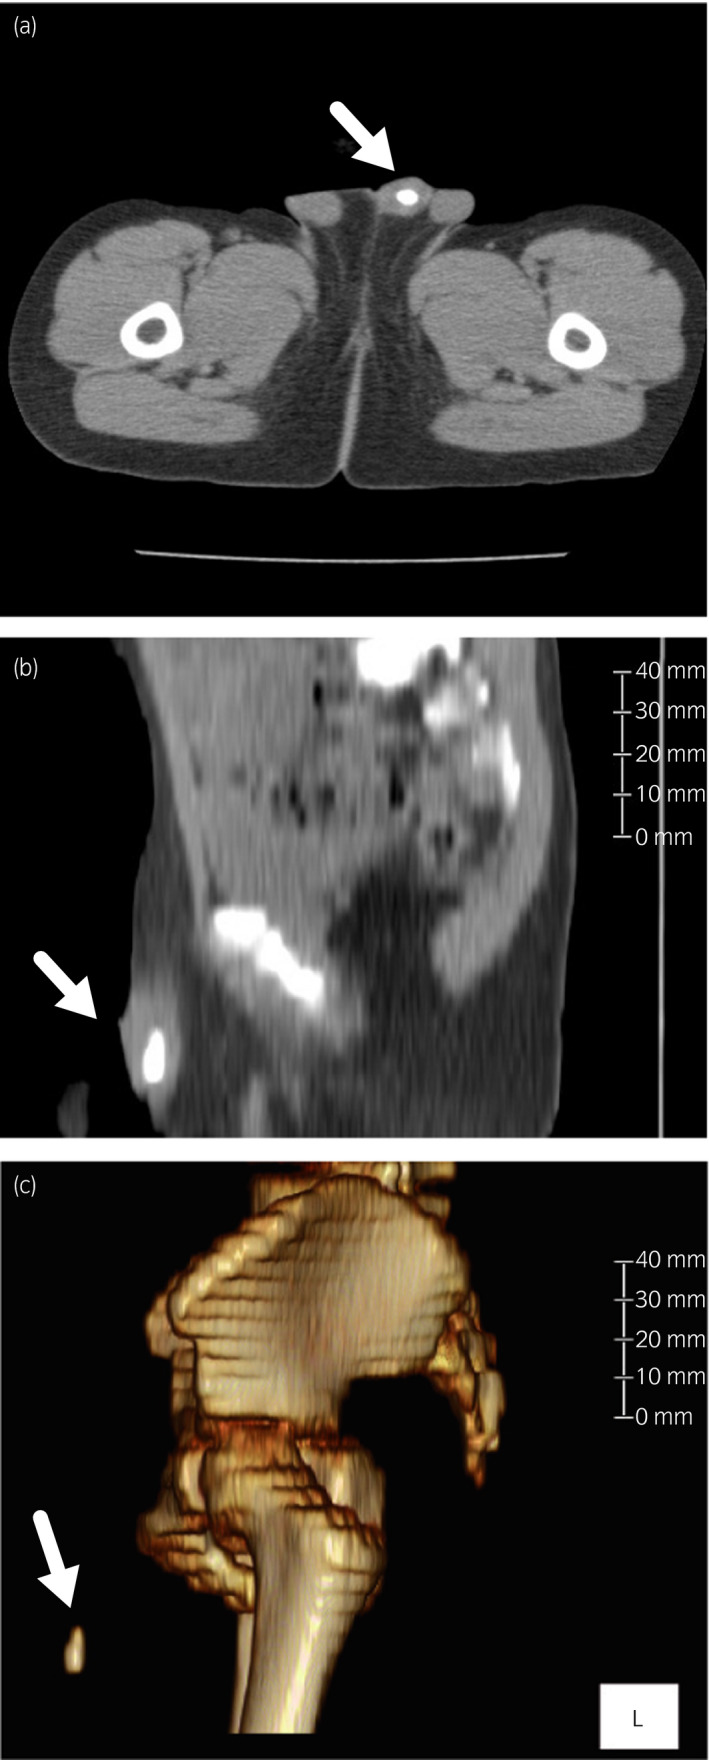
(a) Computed tomography of the urethral stone in the patient. The transverse view reveals a urethral stone (8 × 8 mm). (b) The length of the stone was 1 cm in the sagittal view. (c) The three‐dimensional reconstruction shows a droplet‐shaped stone.
